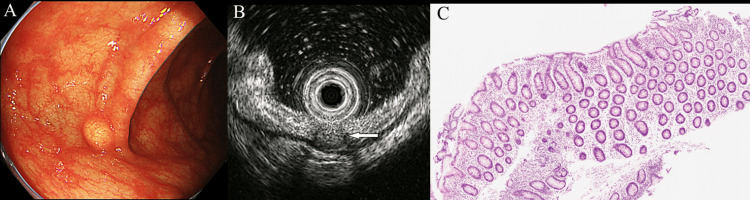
Endoscopic findings. (A) The subepithelial lesion (SEL) appeared yellow in the transverse colon and did not exhibit epithelial changes. (B) Using miniprobe endoscopic ultrasonography (mEUS), the lesion was characterized as a hypoechoic mass located in the deep submucosal layer (arrow) and superficial muscularis propria with a marginal hypoechoic zone. (C) A deep biopsy was performed from the apex of the SEL; however, a definitive diagnosis was not determined.
